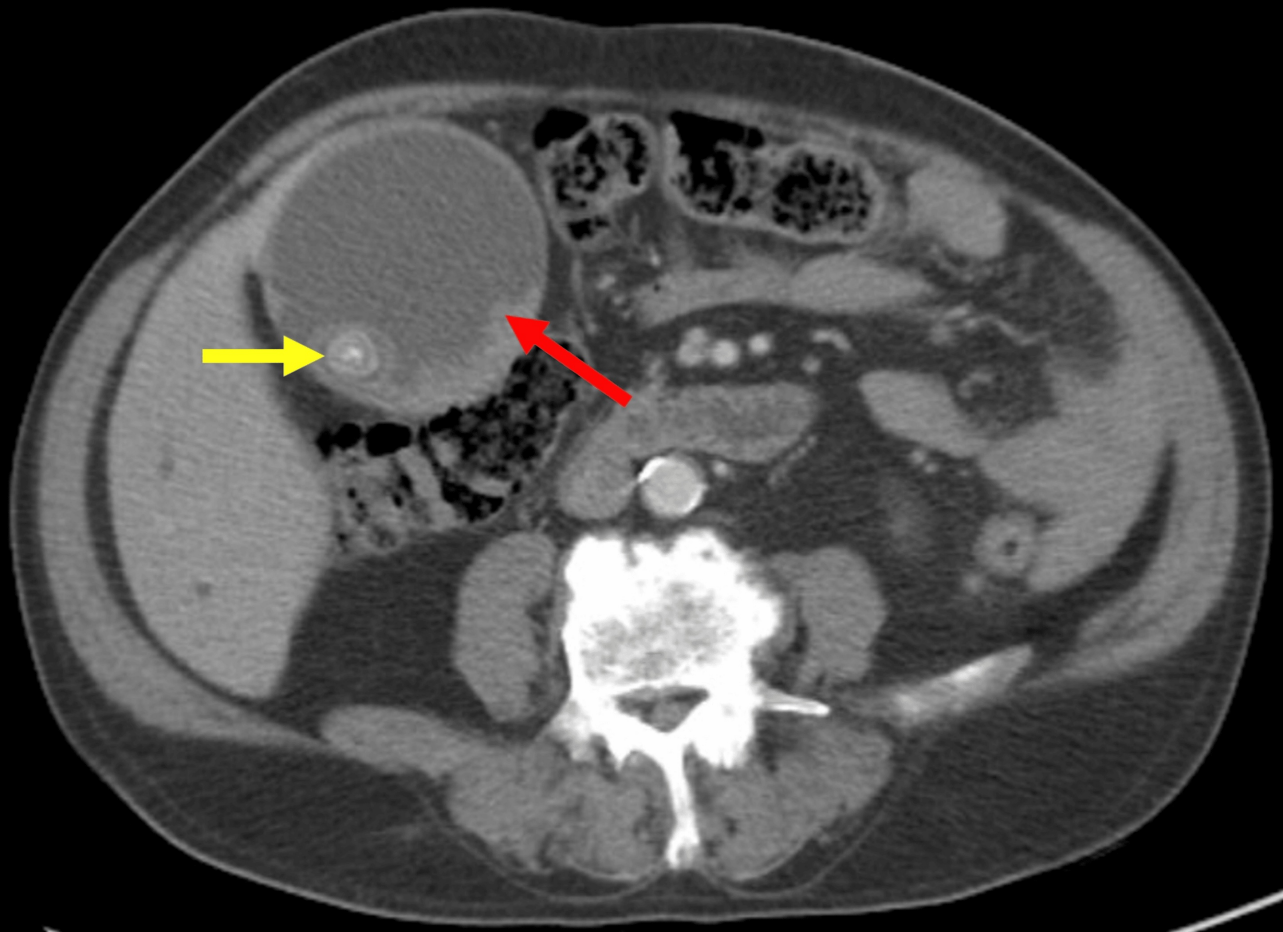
Axial contrast-enhanced CT of the abdomen shows markedly distended gallbladder with irregular wall thickening (red arrow) and intramural density (yellow arrow).
